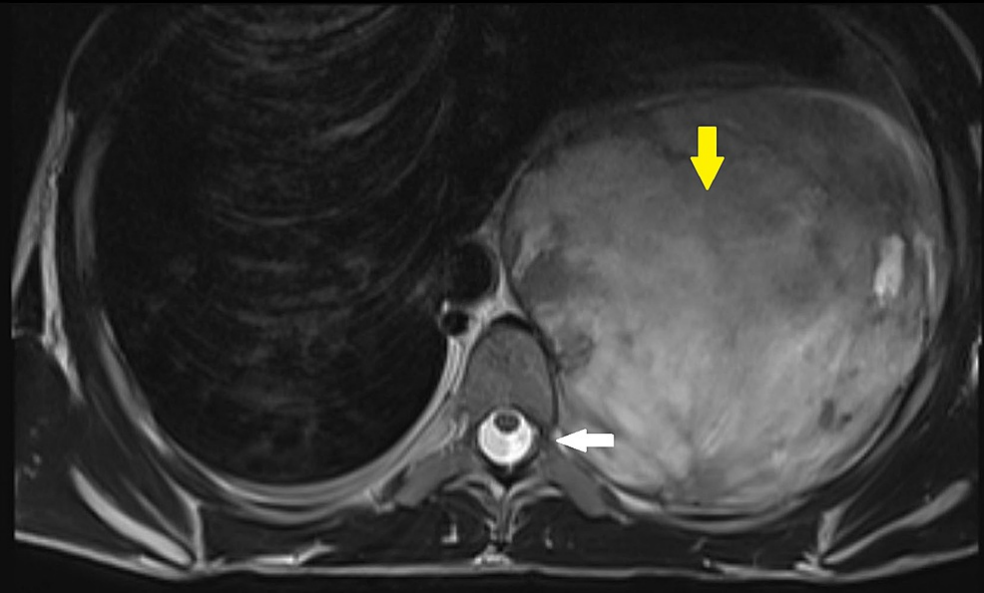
Axial section of T2 weighted MRI shows heterogeneously T2 hyperintense mass lesion (yellow arrow) and no intra-spinal communication was seen (white arrow)
